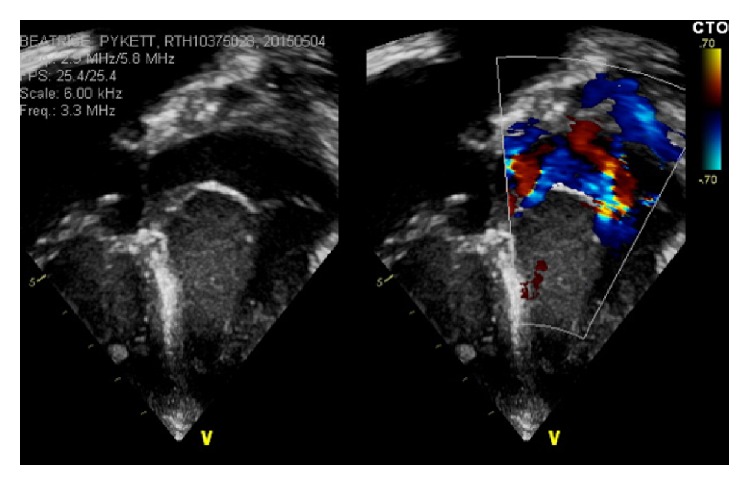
Postsurgical echocardiographic features of the proband: apical 4-chamber view demonstrating reduced mitral regurgitation.
